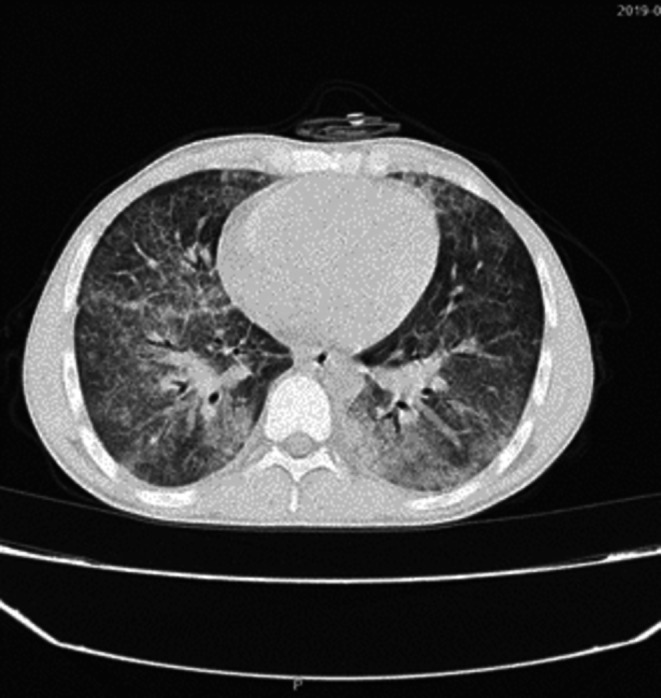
Axial chest CT scan showing bilateral ground‐glass opacities and interstitial thickening.
